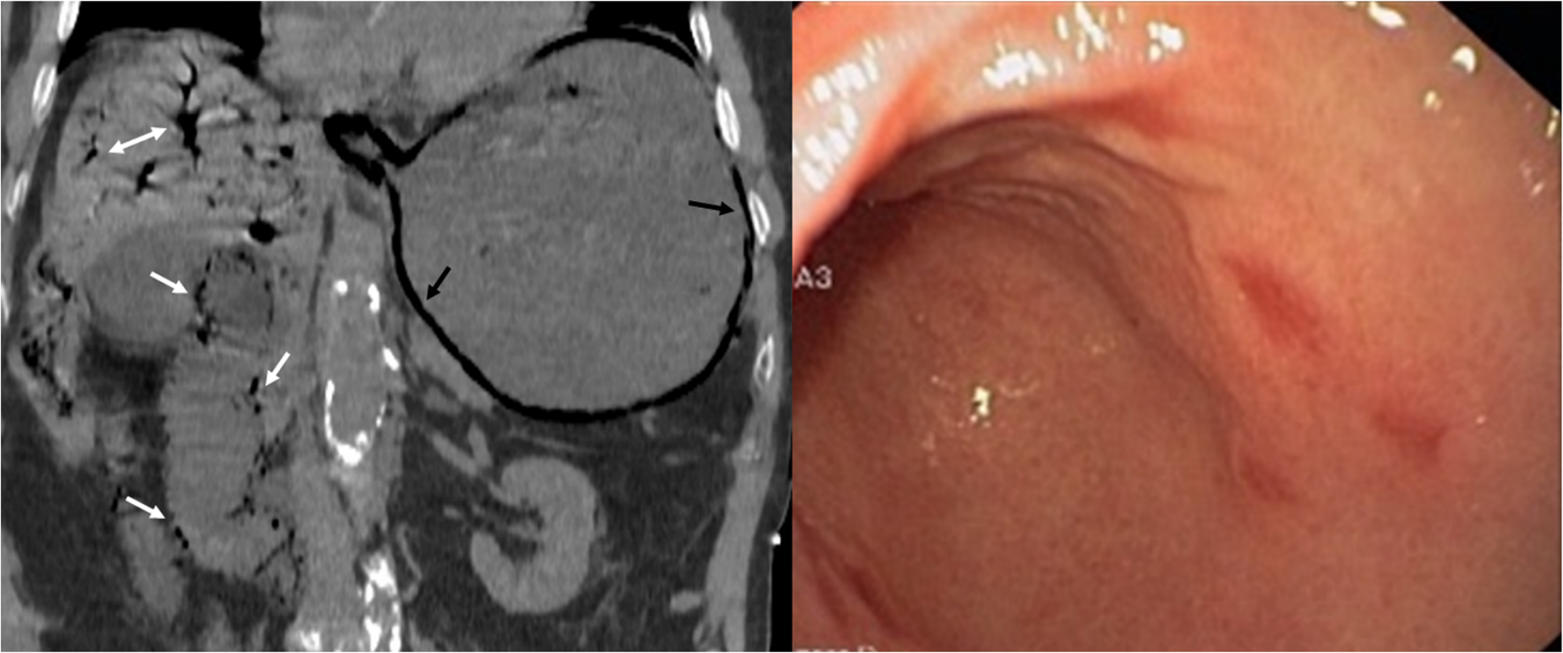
A 58-year-old man with coffee ground vomitus, hypotension, and abdominal pain. Reformatted coronal unenhanced CT image shows a gastroparesis caused by extensive intramural bowel gas (pneumatosis intestinalis) that affects the entire stomach (black arrows) and duodenum (white arrows). Also note gas in the portal vein (double arrow). Upper endoscopy shows a necrotic gastric and duodenal mucosa
